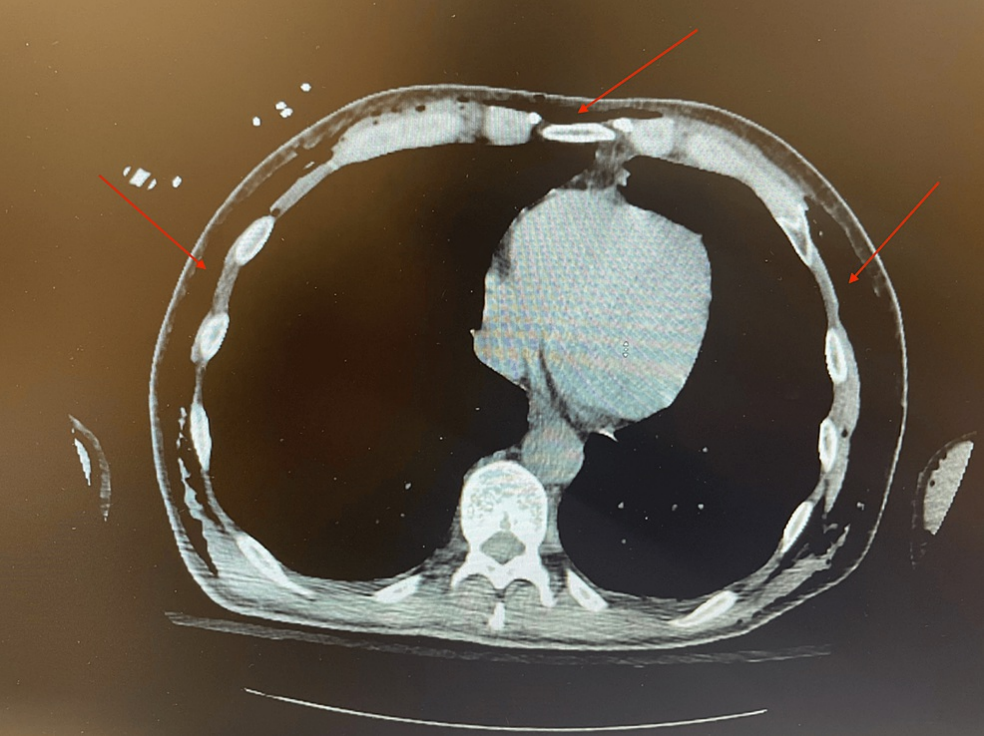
CT scan of the chest obtained postoperatively, which demonstrated extensive soft tissue air in the mediastinum without defects in the tracheal or esophageal wall.
